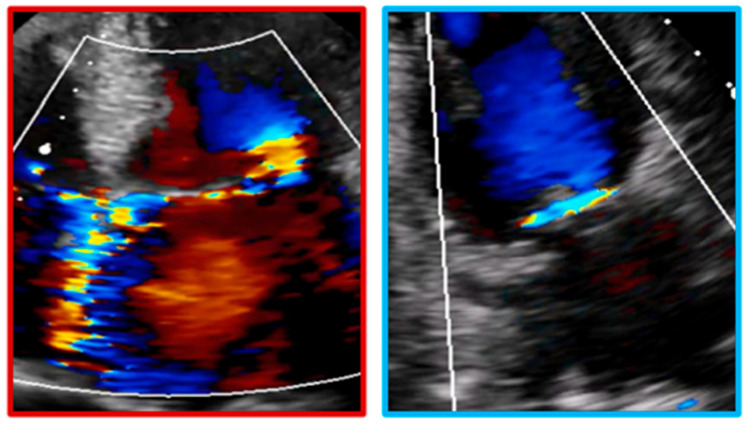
Representation of the “Coanda Effect” and “splay sign”. The first (red panel—Coanda Effect) is displayed in an A4Ch view with an eccentric jet directed towards the interatrial septum. The second (blue panel—splay sign) is shown in an apical two-chamber view, just above the coaptation zone.
